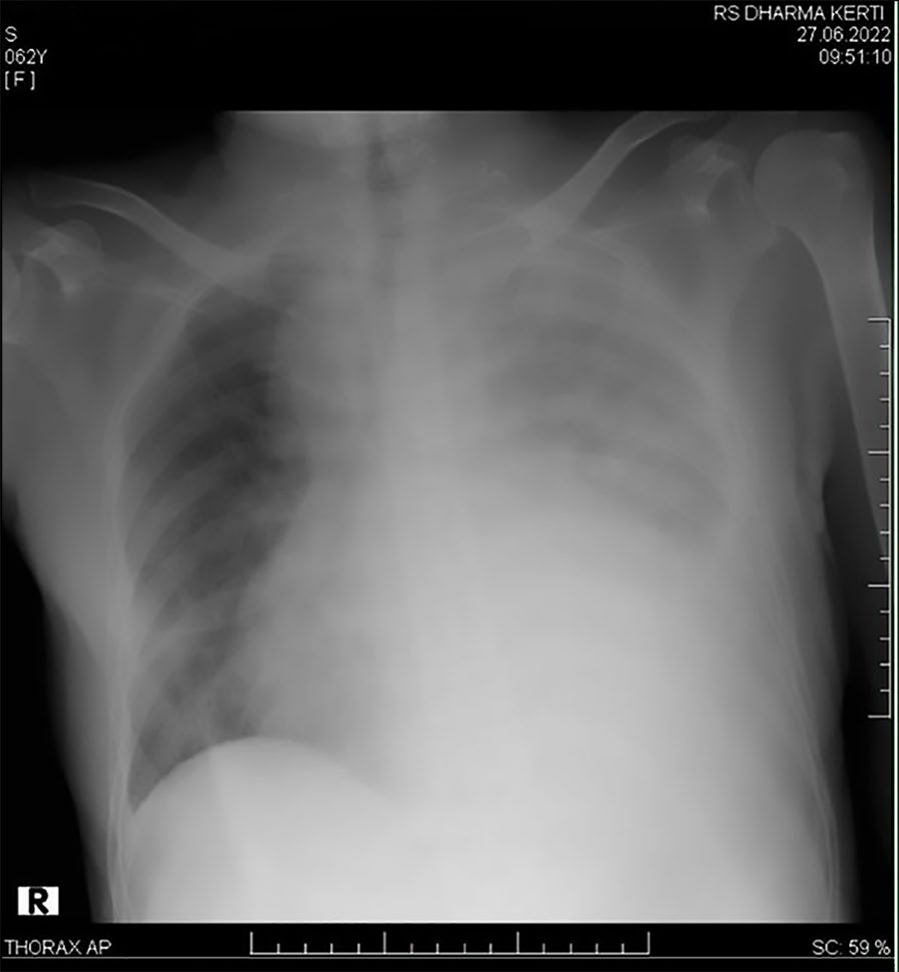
Chest radiograph showed left massive pleural effusion
